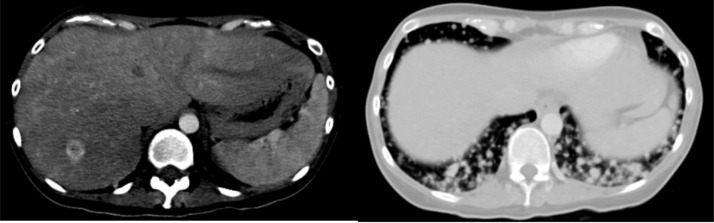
Contrast-enhanced CT scan of the abdomen and lung bases. (Right) There is an arterial enhancing nodule at segment VII of liver, suggestive of a vascular liver metastasis. (Left) Numerous lung nodules in included lung bases in keeping with extensive lung metastases.
